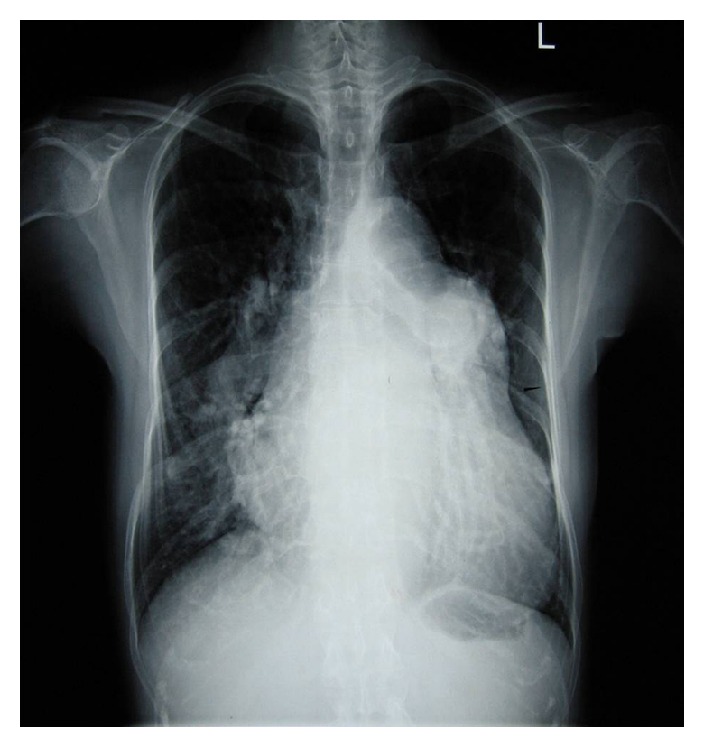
Plain radiographic features of pulmonary artery dissection. Posteroanterior chest radiograph showing cardiac enlargement and pulmonary arterial overcirculation. Pulmonary arterial overcirculation is indicated by prominent pulmonary arterial segments and greatly dilated hilar vessels.
